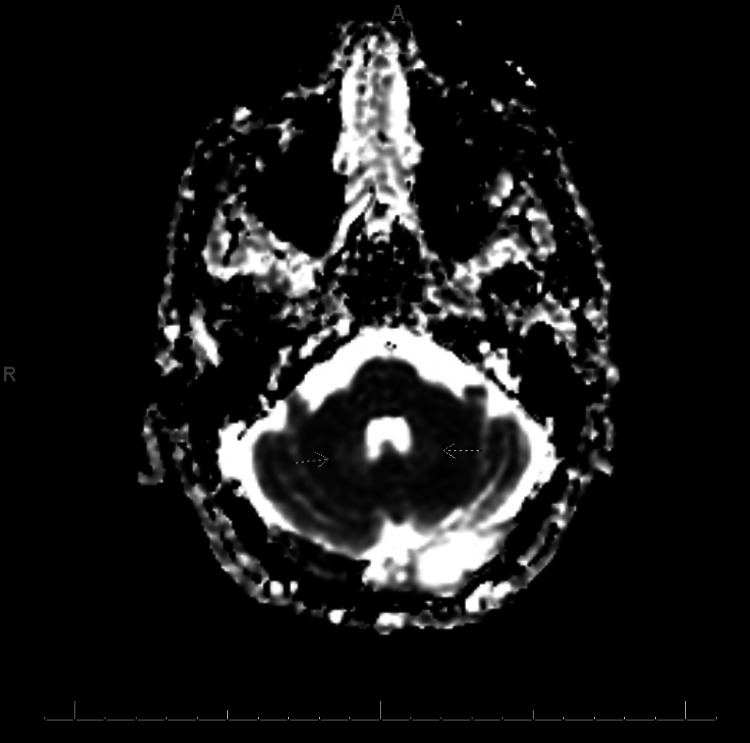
ADC Sequence Brain MRI. Brain MRI of this patient revealed T2 FLAIR bilateral hyperintensity in the dentate nuclei of the cerebellum (Figure 1) along with a bright signal on ADC (arrows on Figure 3).
